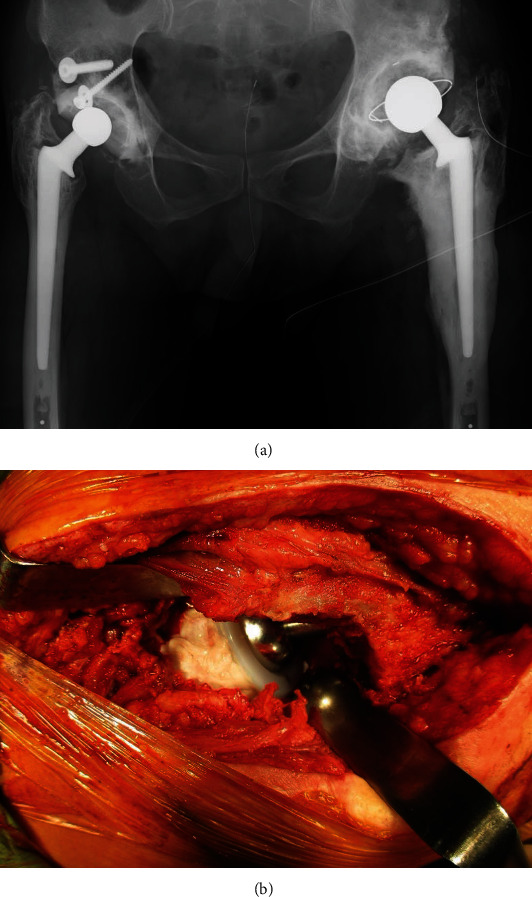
(a) Plain radiograph obtained immediately after the revision THA (second THA) in a 78-year-old man. Abbreviations: THA: total hip arthroplasty. (b) Owing to limited surgical field for a joint revision procedure in a 78-year-old man, using the standard approach because of hip ankylosis, the cement and polyethylene liner were placed onto the acetabulum after impaction of the new head.
